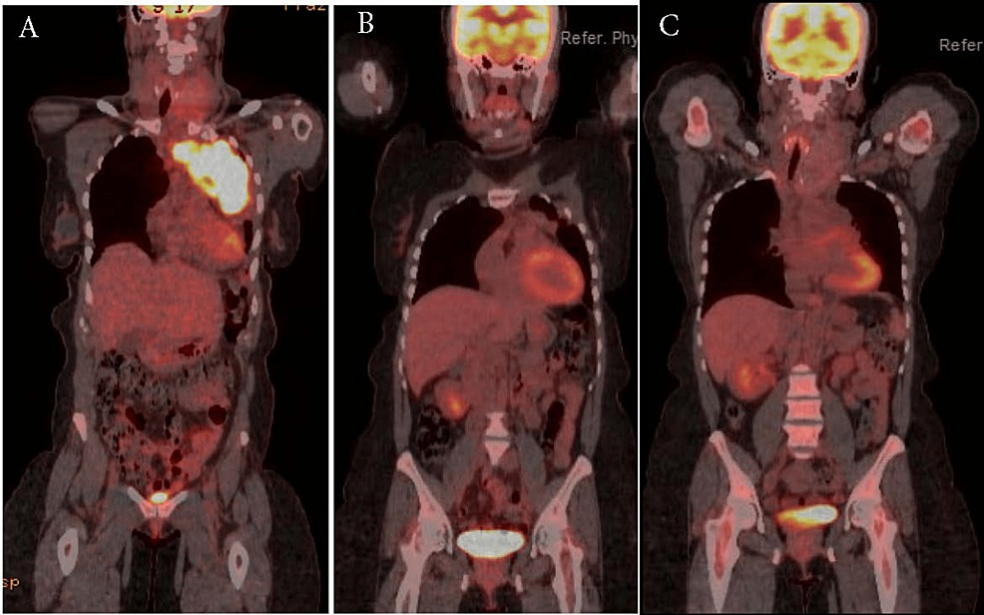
PET-CT of the UMMC patient before and during the IchT. PET-CT = positron emission tomography-computed tomography; UMMC = University of Mississippi Medical Center; IchT = immunochemotherapy
